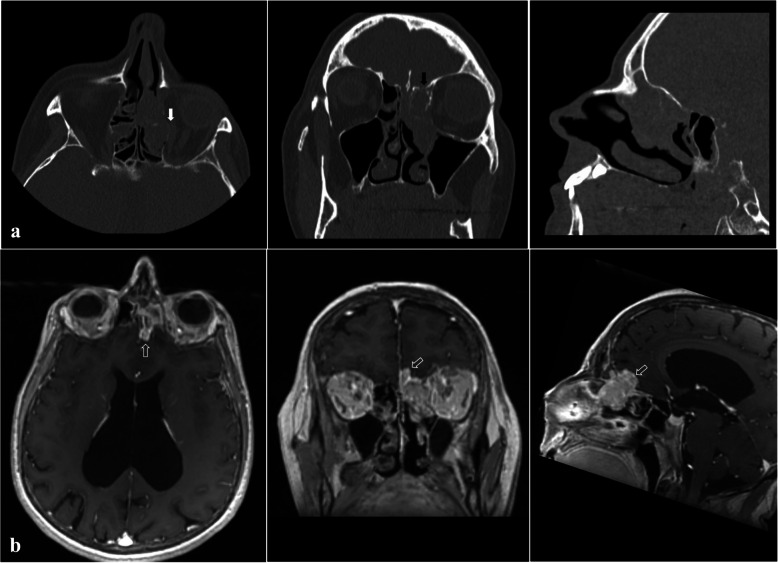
Soft tissue mass involving the fronto-ethmoid area, abutting the left rectal medius muscle (white arrow) with possible intracranial tumor spread (black arrow) visible on a CT scan (a). MRI confirmed the intracranial invasion of the tumor (white empty arrow) (b)
